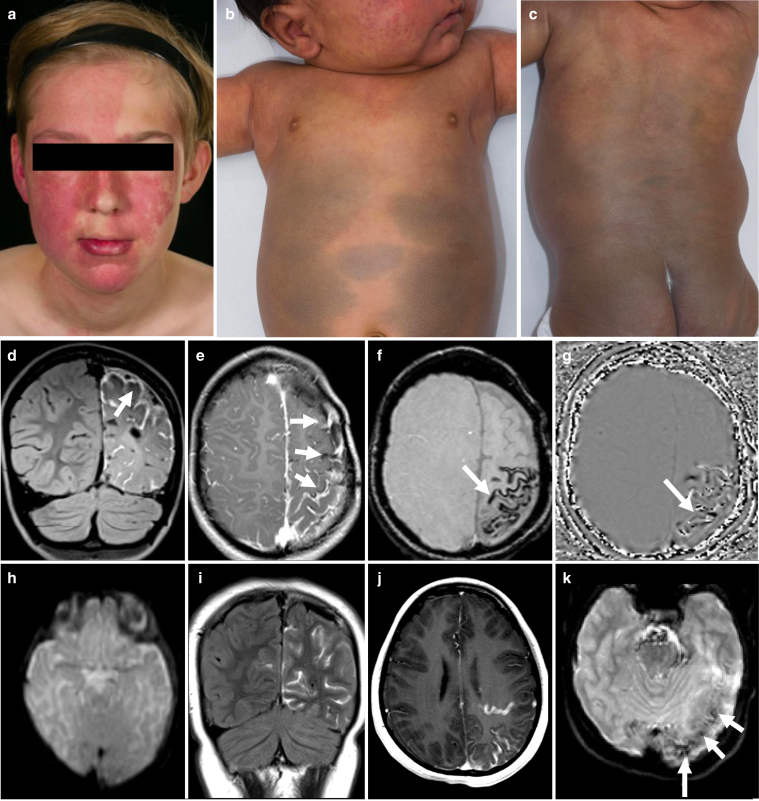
Phenotypic and radiological features in *GNAQ/GNA11* mosaicism. (a) Clinical features of a patient with SWS with a capillary malformation of the face involving the critical forehead area (Waelchli et al., 2014), associated with glaucoma in the right eye. This patient had hypocalcemia with low levels of ionized and total calcium and increased PTH. (b and c) Clinical features of a patient with PPV-DM exhibiting a capillary malformation (with naevus anaemicus) on the face, upper trunk, and extensive areas of dermal melanocytosis. (d and e) Contrast enhanced fluid-attenuated inversion recovery and T1-weighted magnetic resonance images of a patient with SWS show left frontal, parietal, and occipital pial angiomatosis (vascular malformation, arrows). (f) Susceptibility-weighted imaging (SWI) and (g) SWI phase map depicting vascular calcifications (arrow). This patient had low levels of ionized calcium and total calcium with PTH in the normal range. (h) Axial DWI b0 image does not show calcifications on the baseline magnetic resonance imaging study. (i–k), images from 8-years later. (i) Coronal fluid-attenuated inversion recovery after administration of a gadolinium-based contrast agent shows left parietal and occipital (and some contralateral) sulcal enhancement in keeping with pial angioma. (j) Axial postcontrast T1-weighted image depicts the sulcal enhancement and a prominent draining vein. (k) DWI b0 image at follow-up shows foci of increased susceptibility which are suggestive of calcification. Patients and/or parents/guardians provided written consent for the publication of images. Ca/Cr, calcium to creatinine ratio; PTH, parathyroid hormone.
